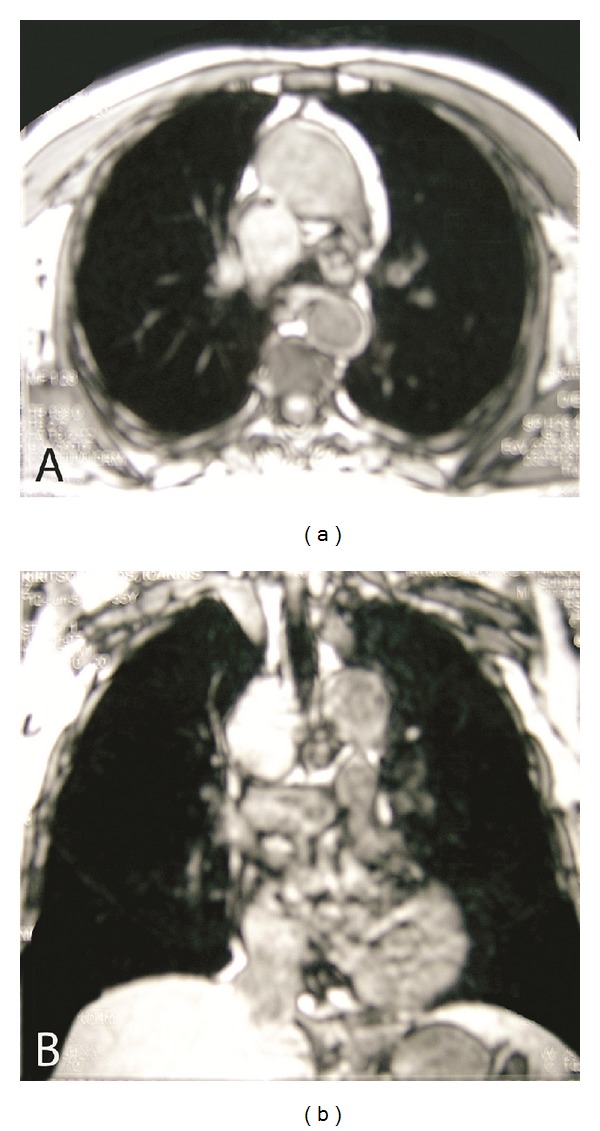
Axial (a) and coronal (b) chest MR images demonstrate right paratracheal lymph node mass.
